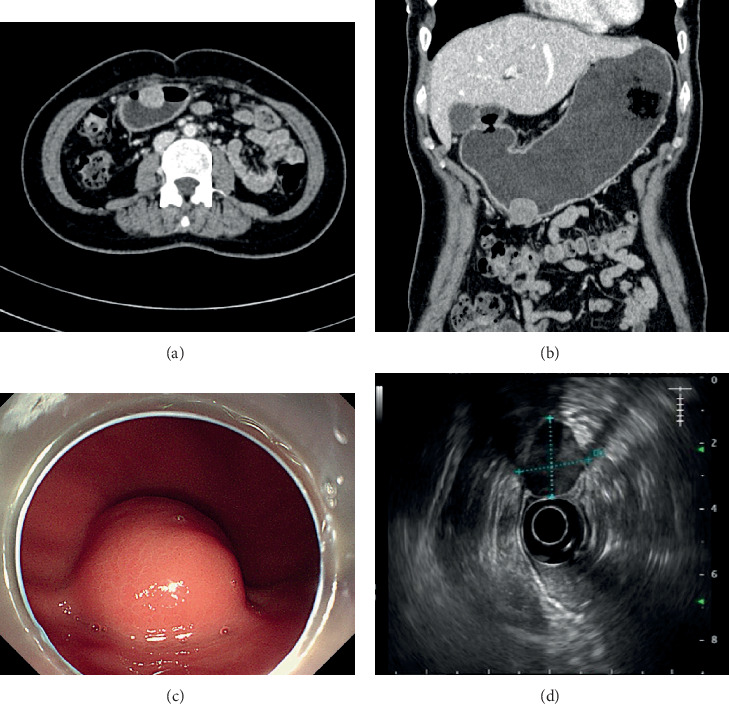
Characteristic findings of imaging modalities in patients with gastrointestinal schwannomas. (a, b) Computed tomography scan revealing a round, well defined, soft-tissue density tumor with moderate enhancement in axial plane (a) and coronal plane (b). (c) Endoscopy showing a domed submucosal lesion with intact overlying mucosa. (d) Endoscopic ultrasonography showing a hypoechoic mass on the gastric wall.
